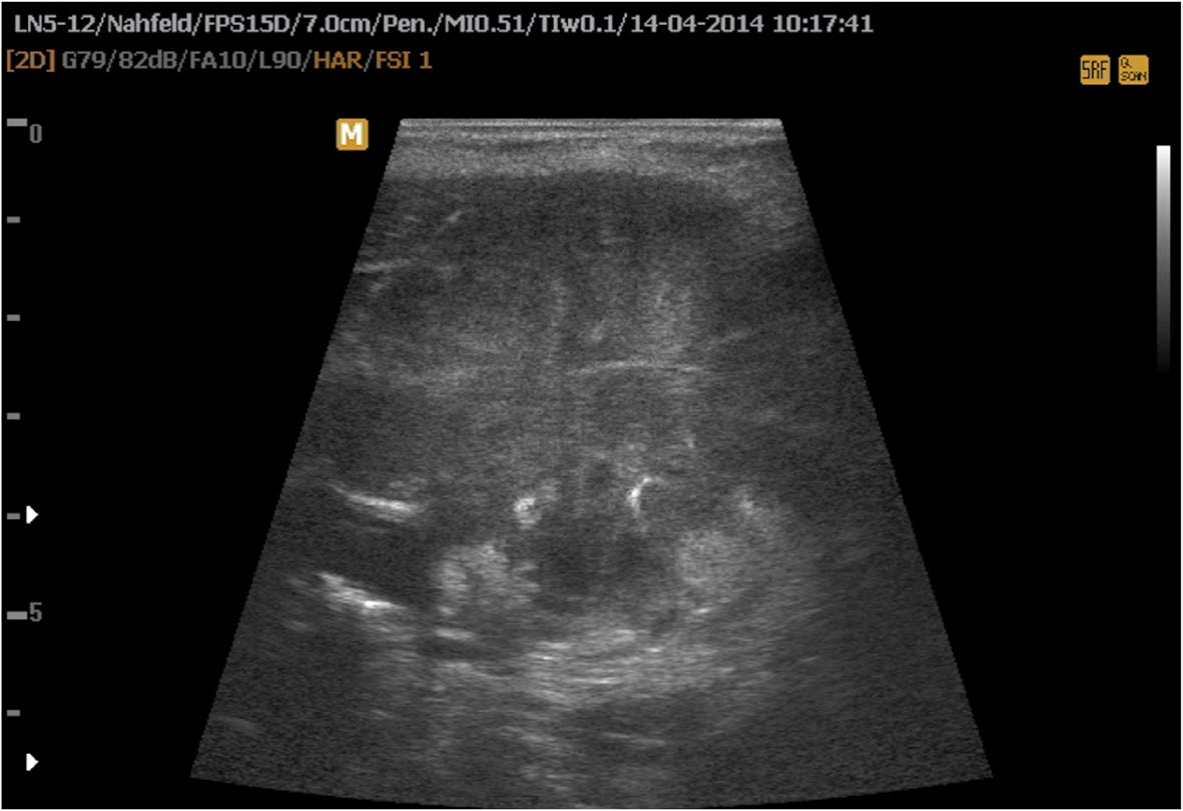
Ultrasound imaging: large left sided mass with inhomogeneous tissue, ill defined borders, and infiltration of muscle. For a video clip see supplementary file
